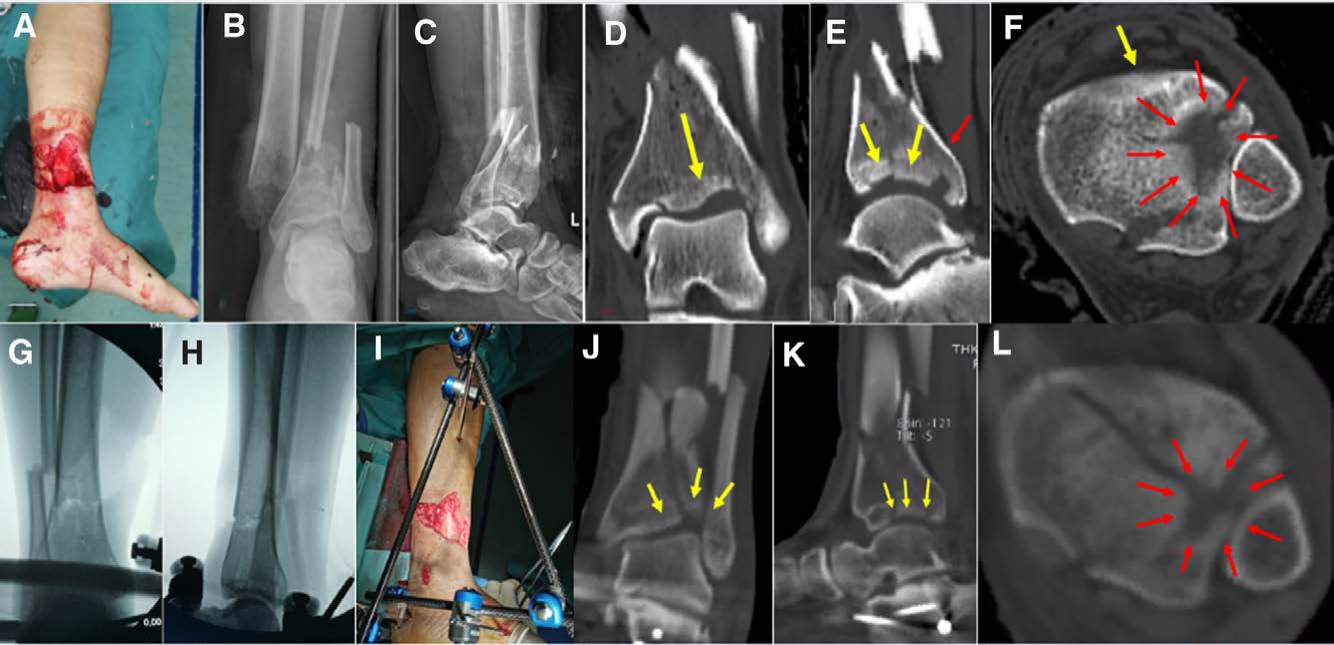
The left ankle in case 2 had an open fracture with the broken end exposed (A). The X-ray showed a comminuted fracture of the left distal tibiofibular (B and C). CT scan showed a comminuted fracture of the left distal tibia and fibula with collapse of the central articular surface of the distal tibia and an intact anterior cortex (D–F). The broken end of the fracture was fixed with an external fixator during surgery, and fluoroscopy confirmed good recovery of the force line (G and H). Postoperative CT showed that the articular surface was partially repositioned, but the central region was still partially collapsed (J–L). CT = computed tomography.
